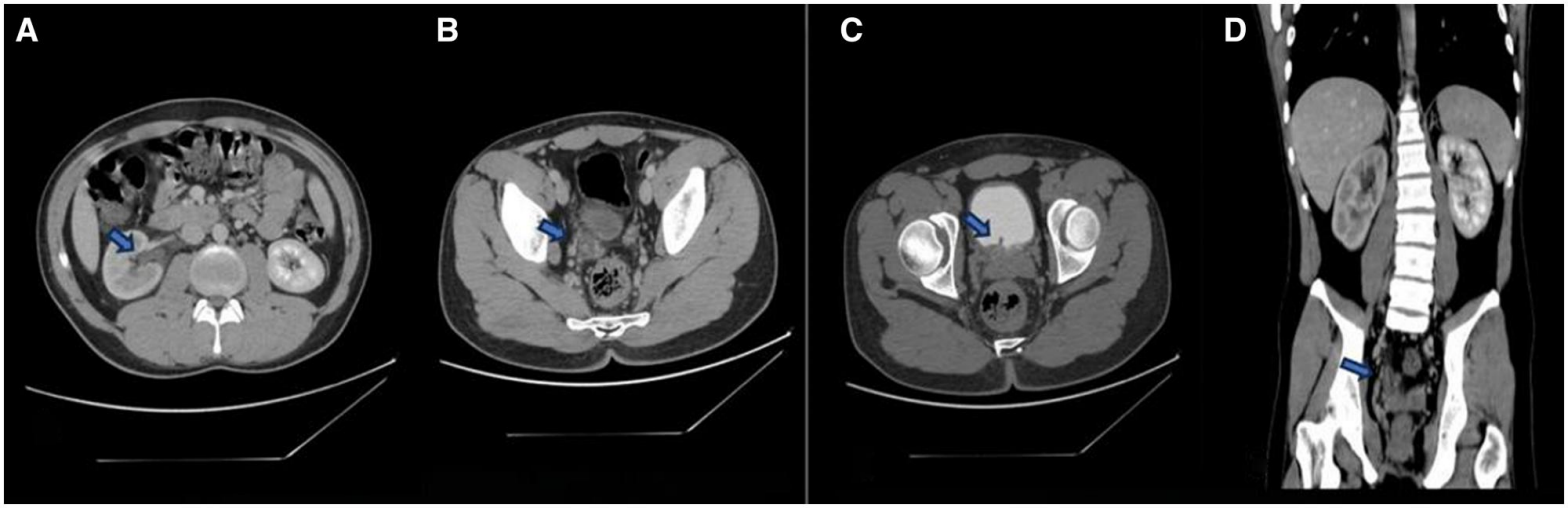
(A) Axial image at a level right renal pelvis on venous phase showing mild hydronephrosis abdominal perinephric fat stranding due to the distal ureteric obstruction it also shows hypo enhancement of the right kidney compared to the left side. (B) Axial image at the level of the distal part of the right ureter showing dilated ureter and rounded filling defect representing the obstructing parasite. (C) Axial CT image at the level of VUJ at 10 min delay phase showing the parasite partially passing through the vesicoureteral junction. (D) Coronal CT image showing the cylindrical structure at the distal ureter.
